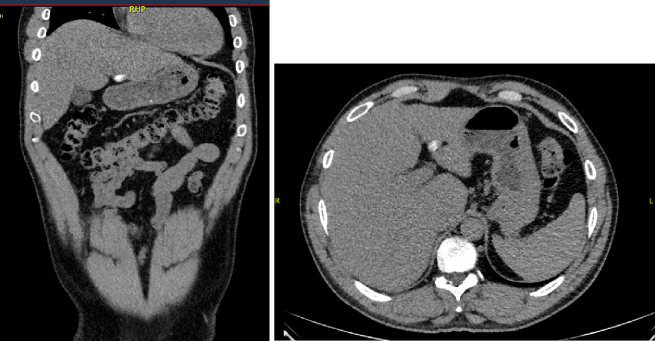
CT showing calcified cyst in segment III of the liver.
